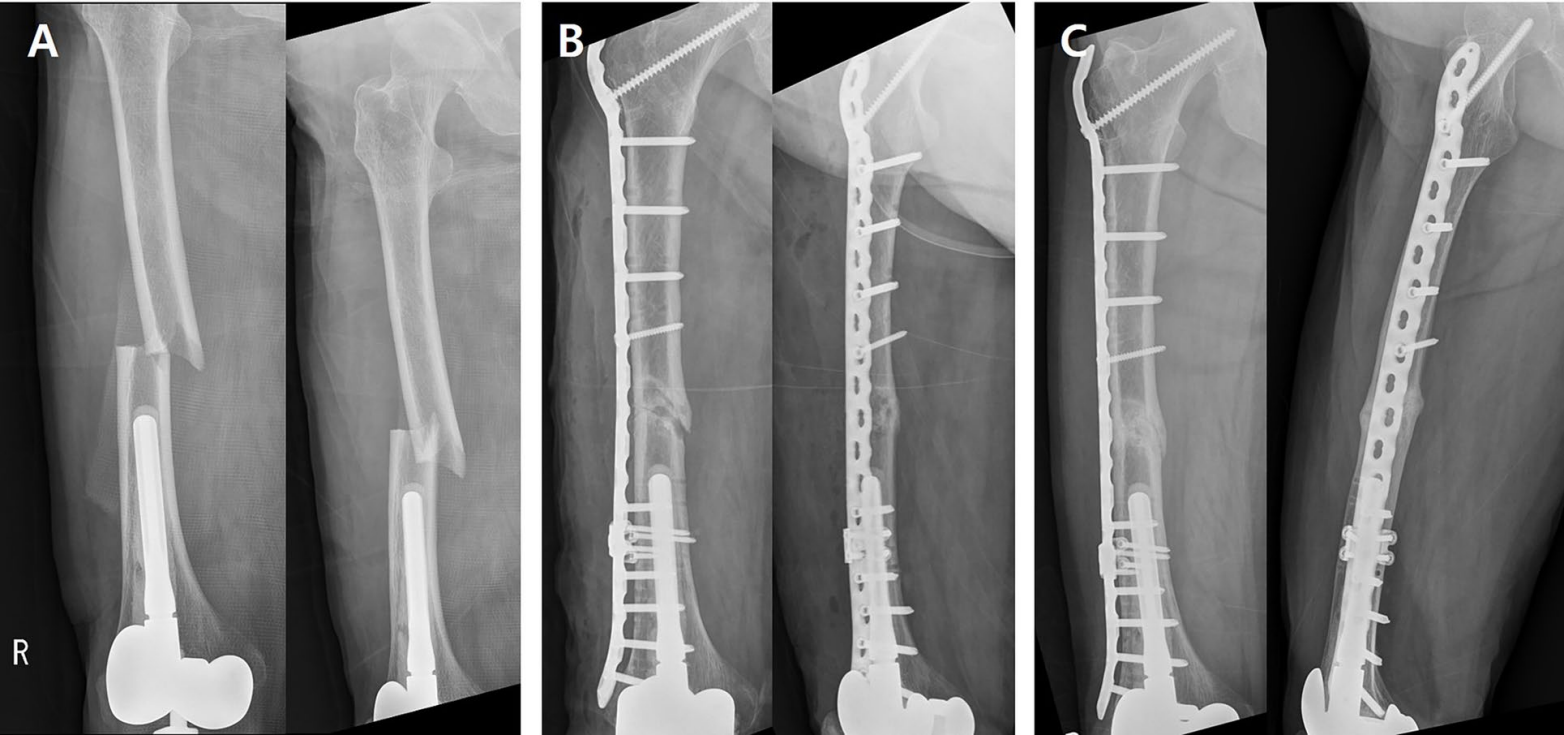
A Preoperative anteroposterior and lateral plain radiographs of an 83-year-old female who sustained a periprosthetic femoral shaft atypical fracture following TKA with long stem, representing a Su type I. B The patient was treated with a 4.5-mm LCP long broad-curved plate overlapping the zone of the stem at least 6 cm. Intraoperative plate bending was performed using a large bender to fit the proximal and distal femoral geometry. Supplementary fixation using a LAP was performed in the overlapped zone. Fixation of the femoral neck was additionally performed using a 4.5-mm long cancellous screw to protect the entire femur from future fracture. C At 4 months postoperatively, a solid bone union was acquired. TKA, total knee arthroplasty; LCP, locking compression plate; LAP, locking attachment plate
